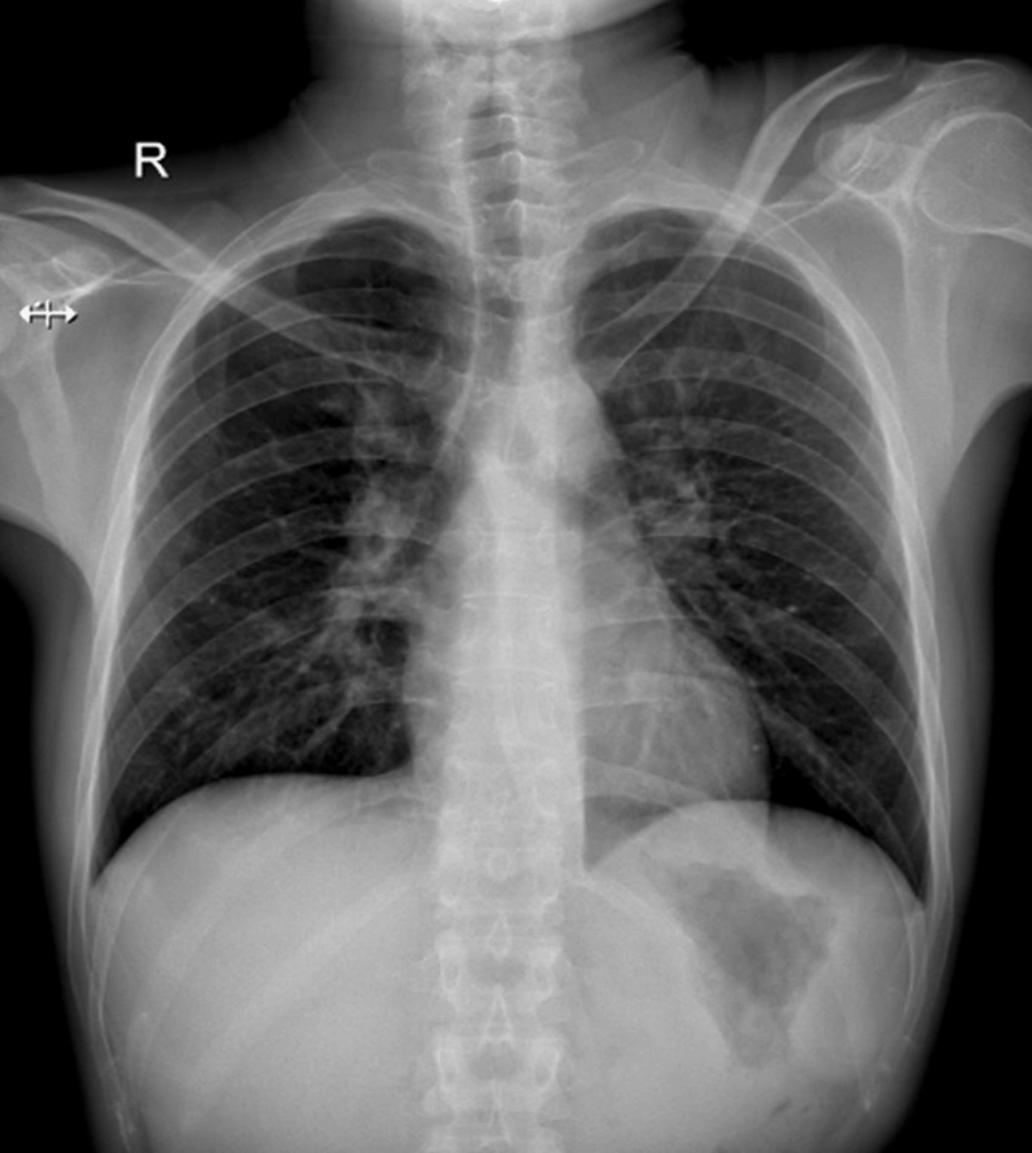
Plain chest radiograph showing near complete resolution of bilateral hilar opacities, post 4 months of steroid treatment.
